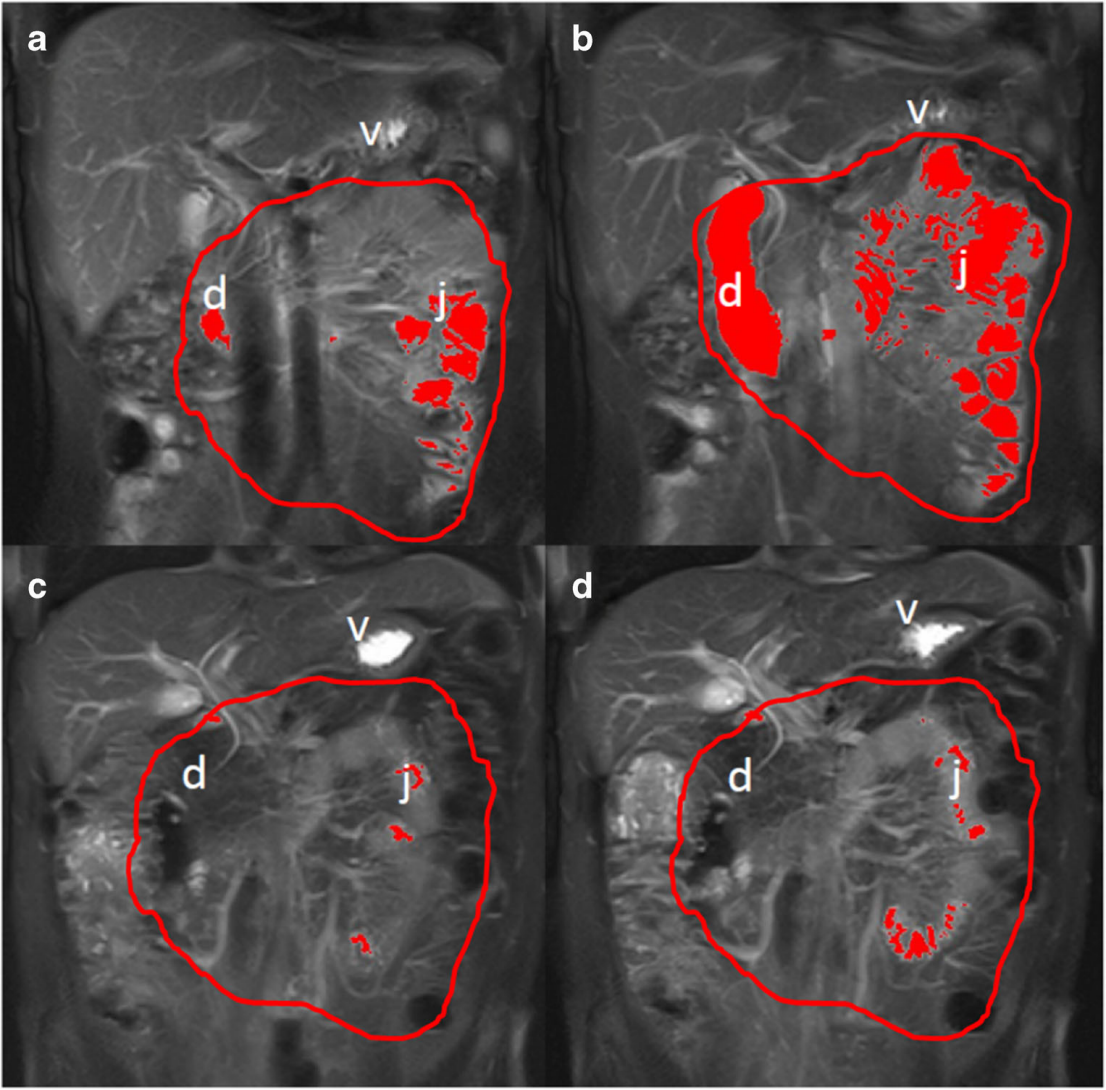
T2-weighted MRI images before (A, C) and 13 minutes after secretin stimulation (B, D) with hyper-intense fluid signal segmented in red within defined region of interest comprising the duodenum and jejunum (boundaries marked with *red*). The *upper panels* demonstrate a pancreas-insufficient patient, whereas the *lower panels* demonstrate a pancreas-sufficient CF patient. d: duodenum, v: ventricle, j: jejunum
